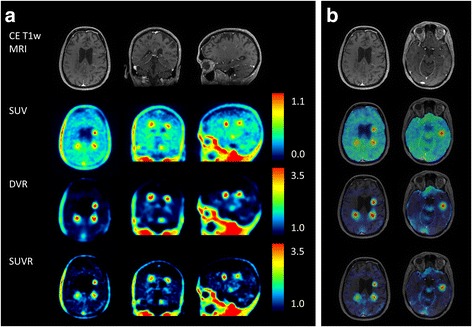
Images of an MS patient in native [^18^F]GE-180 PET space. Top row: T_1_-weighted contrast-enhanced MRI. Second row: SUV image (60–90 min p.i.). Third and bottom row: DVR (from dynamic data reconstructed with 10-mm Gauss filter) and SUVR with lower threshold set to 1.0 for the depiction of specific binding relative to the PRR. a Whole head in triangular views. b Application of brain mask onto parametric maps for two different transaxial planes with MRI overlay
